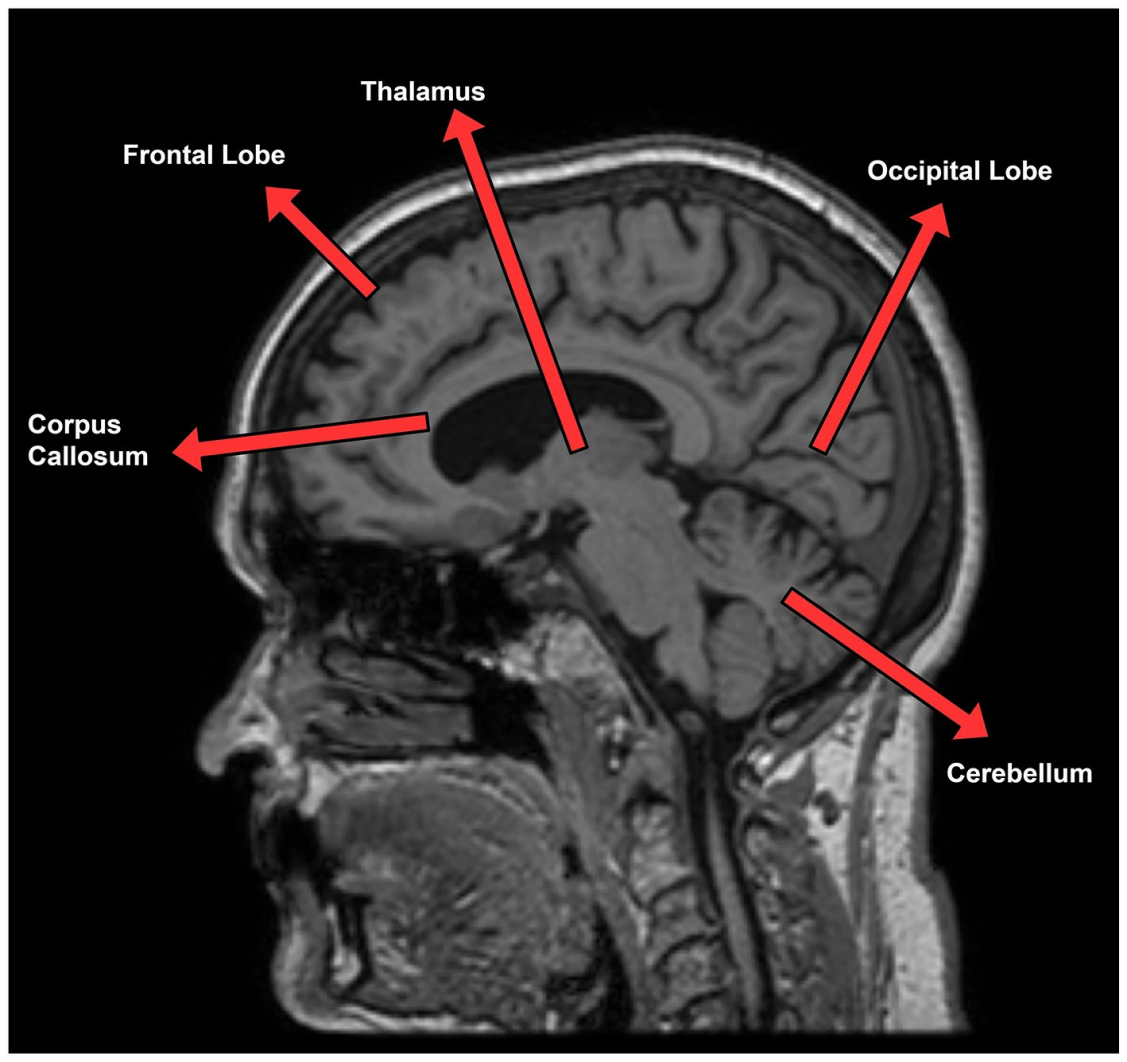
Sagittal plane view of MRI scan taken from ADNI dataset describing AD relevant section of the brain.
